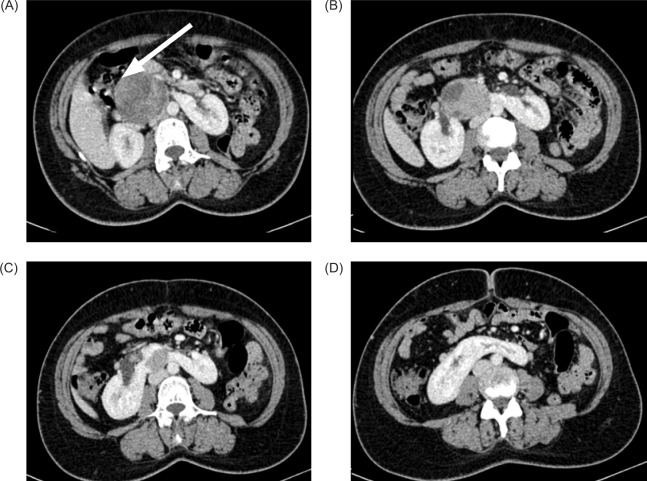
(A–D) Axial contrast enhanced CT abdomen showing well-defined heterogeneously enhancing solid mass arising from isthmus compressing and displacing adjoining second and proximal third portion of the duodenum (arrow in [A]).
